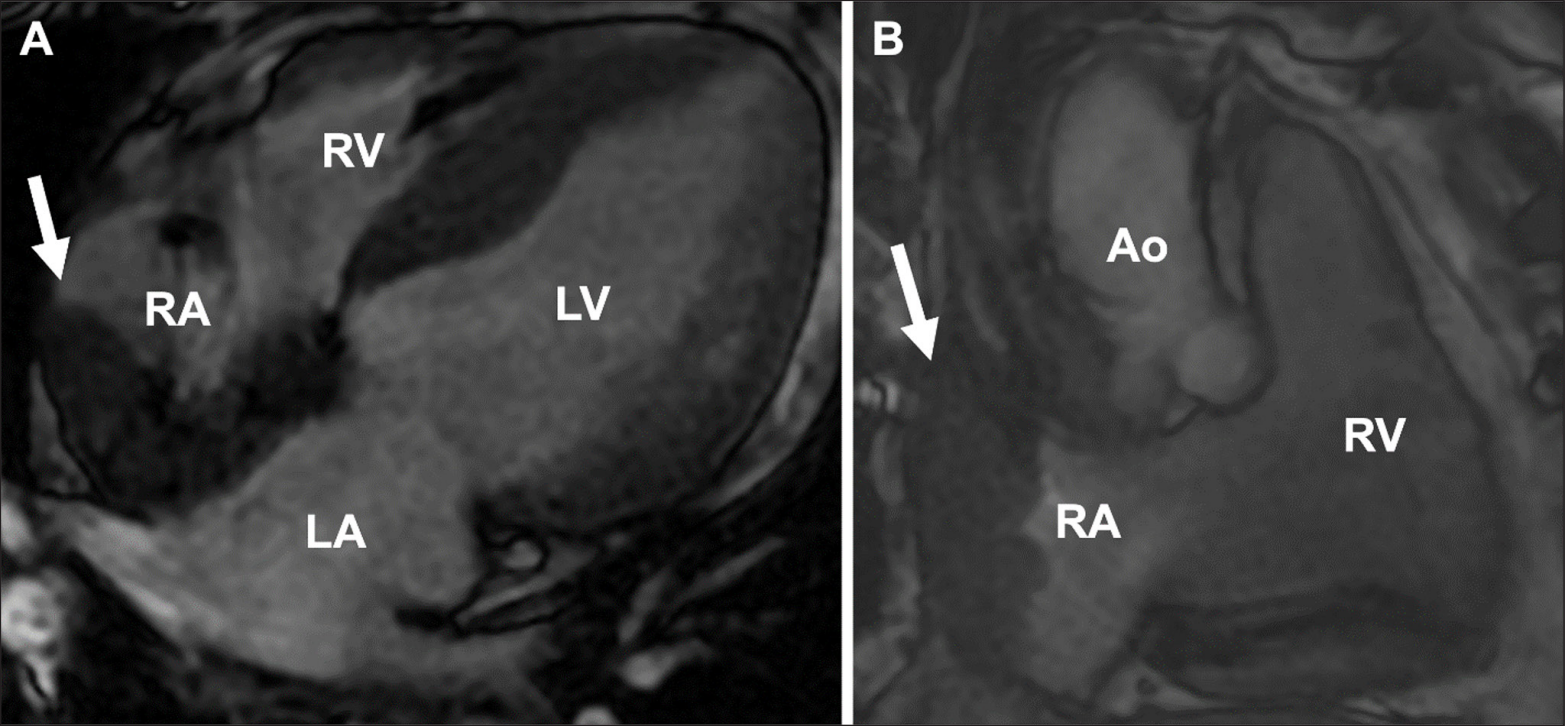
(A) Gadolinium cardiac magnetic resonance imaging in transverse view showing heterogeneous hyperenhancement of right atrial mass (arrow) occupying the right atrium, with right ventricle, left atrium, and left ventricle. (B) Same right atrial mass (arrow) redemonstrated in sagittal view with RV and aorta. RA: right atrium; RV: right ventricle; LV: left ventricle; LA: left atrium; Ao: aorta
